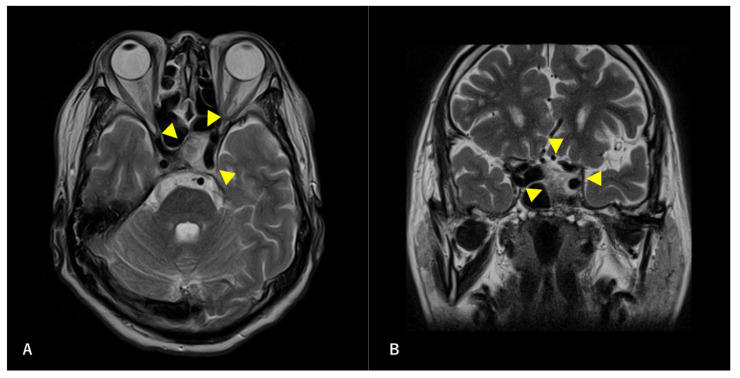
Preoperative T2-weighted magnetic resonance image (MRI) findings. Axial section (A) and coronal (B) images are shown. A high-intensity mass is observed in the left sphenoid sinus. The ICA is surrounded by a mass. Arrowheads indicate tumors.
